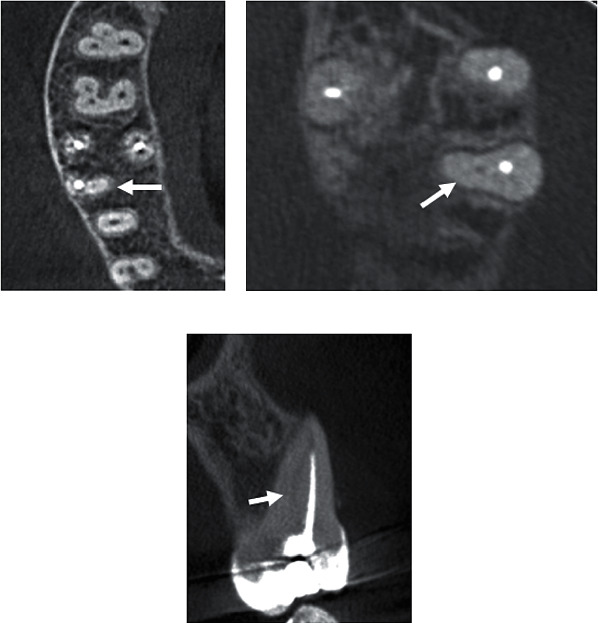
CBCT slices of the maxillary first molar: (a, b) axial section with the missed MB2 canal (arrows); (c) coronal section with the missed MB2 canal (arrow).
